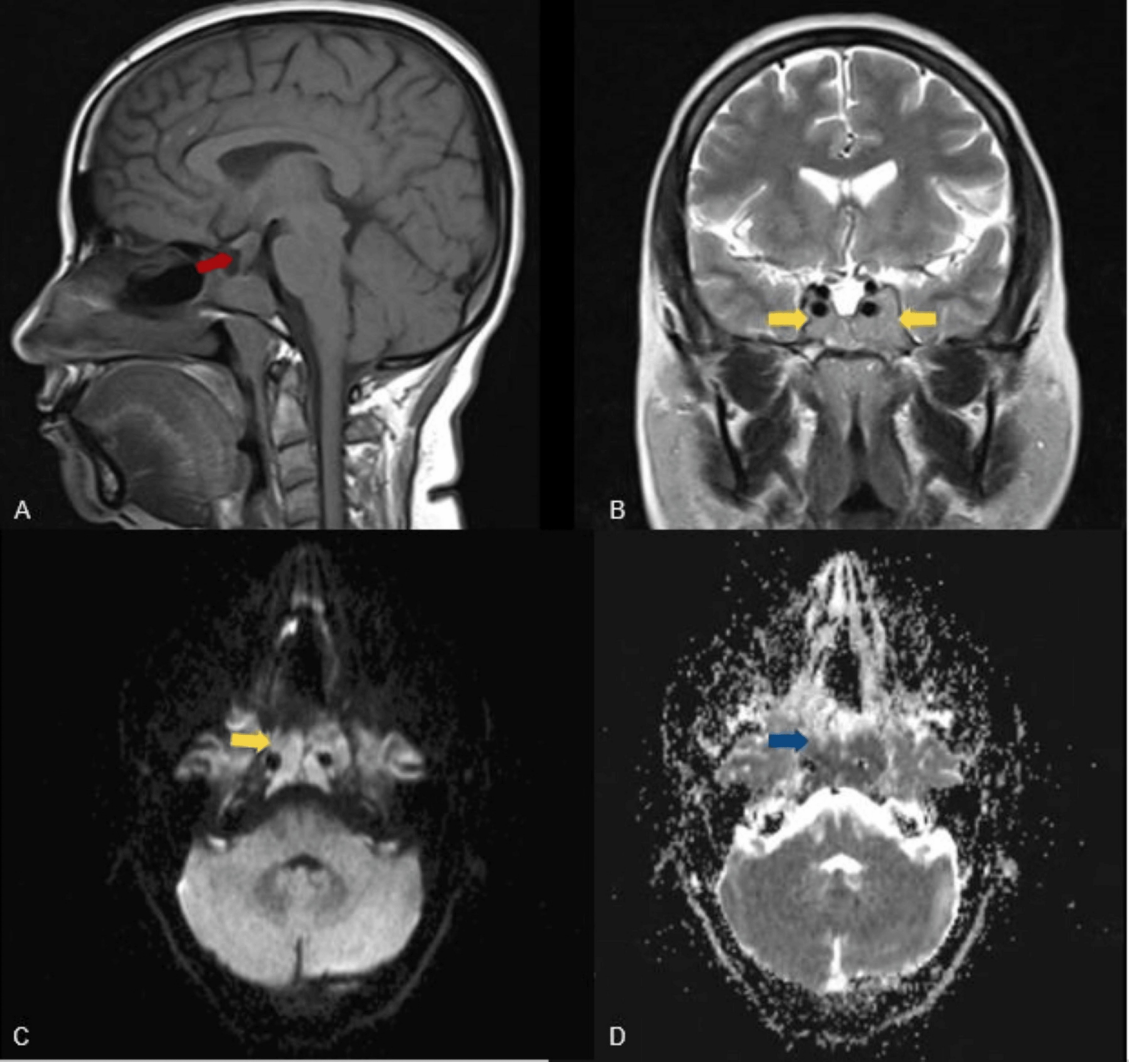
MRI findings - 1 Images showing pituitary macroadenoma involving infundibulum (red arrow) and bilateral cavernous sinuses (yellow arrows), showing diffusion restriction on DWI (green arrow) with low ADC values (blue arrow). A: sagittal T1; B: coronal T2; C: DWI; D: ADC ADC: apparent diffusion coefficient; DWI: diffusion-weighted imaging; MRI: magnetic resonance imaging
